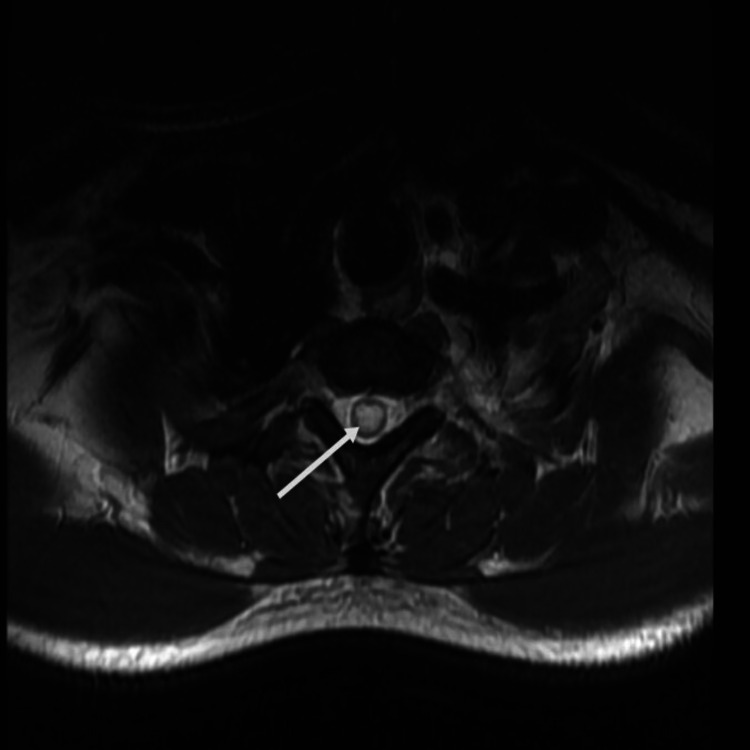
Axial section of T2-weighted MRI showing a central circular hyperintensity within the central canal.
